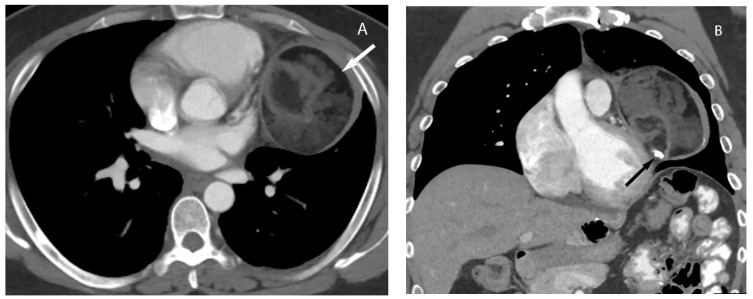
Mature teratoma. CT axial (A) and coronal reformat (B) show a well-defined mass containing soft tissue, fat (arrow in (A)) and calcification (arrow in (B)) in the prevascular mediastinum compatible with mature teratoma.
